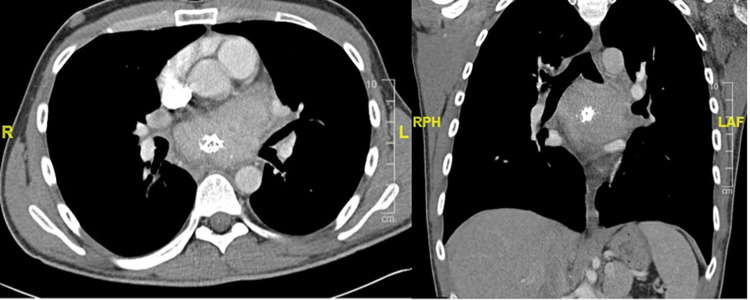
Chest CT demonstrating large mediastinal mass measuring 8.2 cm. Left: axial Right: coronal
